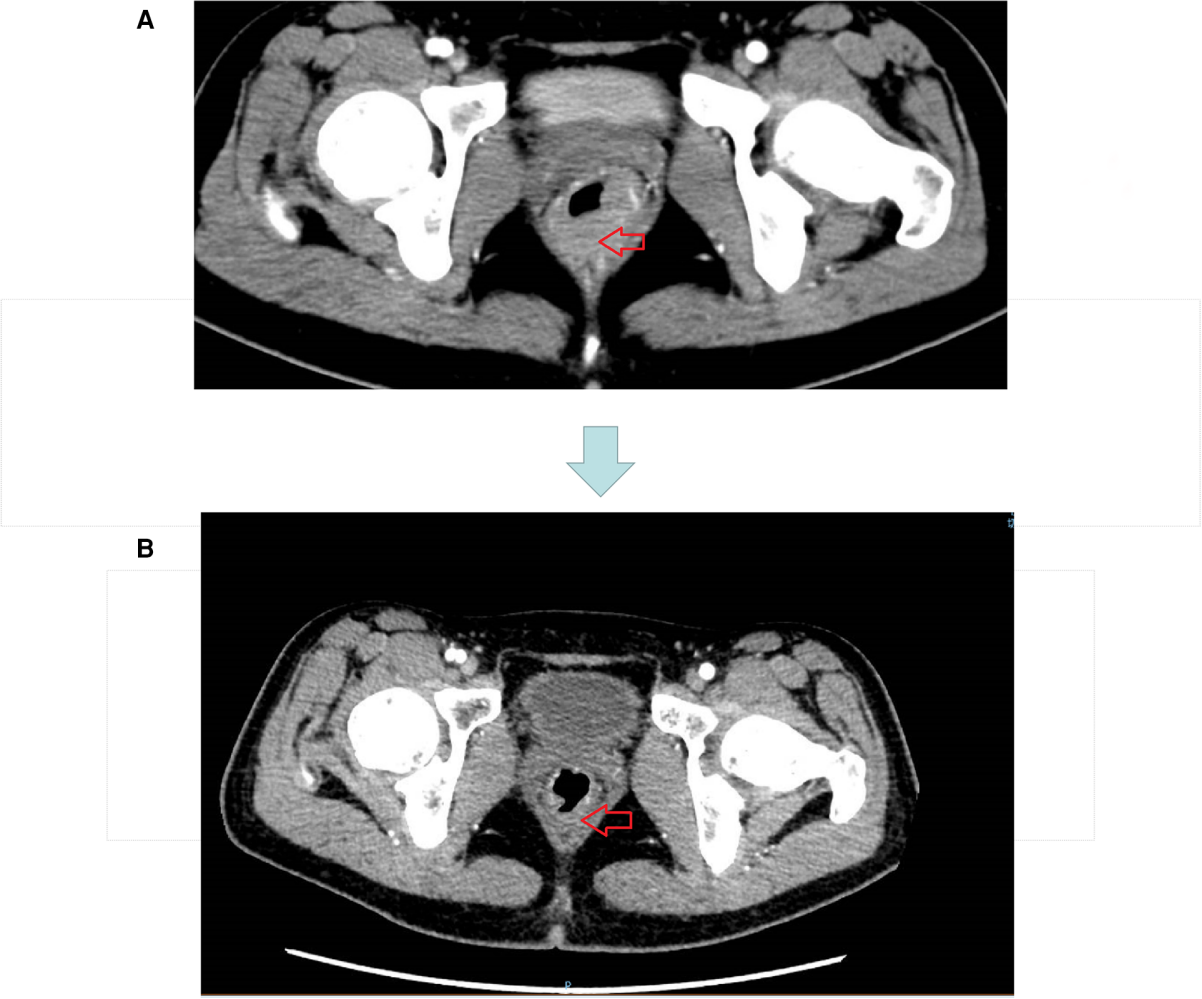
(A) CT image before neoadjuvant therapy. (B) CT image after three cycles of short course radiotherapy + XELOX + camrelizumab.
